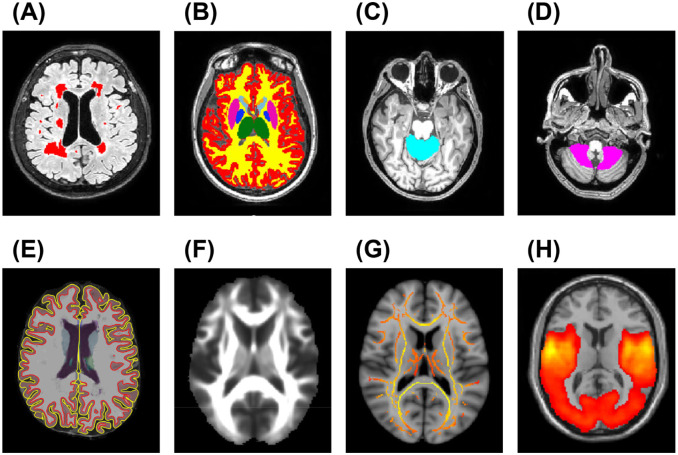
Examples of brain MRI analysis performed in this study. (a) Brain lesion segmentation (red-coded) on co-registered 3D FLAIR and 3D T1-weighted images; (b) segmentation of WM (yellow), cortex (red), and thalamus (green) on lesion-filled 3D T1-weighted images using SIENAx2; (c, d) segmentation of ACMA (light-blue) and PCMA (pink) on lesion-filled 3D T1-weighted images using SUIT; (e) WM surface (red) and pial surface (yellow) obtained using FreeSurfer 7.1.1. on lesion-filled 3D T1-weighted images for calculation of Cth; (f, g) mean FA image and WM tract skeleton obtained during DTI processing; (h) seed-based RS FC network of the left M1 in our cohort. Abbreviations: ACMA = anterior cerebellar motor area; Cth = cortical thickness; DTI = diffusion tensor imaging; FLAIR = fluid-attenuated inversion recovery; M1 = primary motor cortex; PCMA = posterior cerebellar motor area; RS FC = resting-state functional connectivity; WM = white matter.
